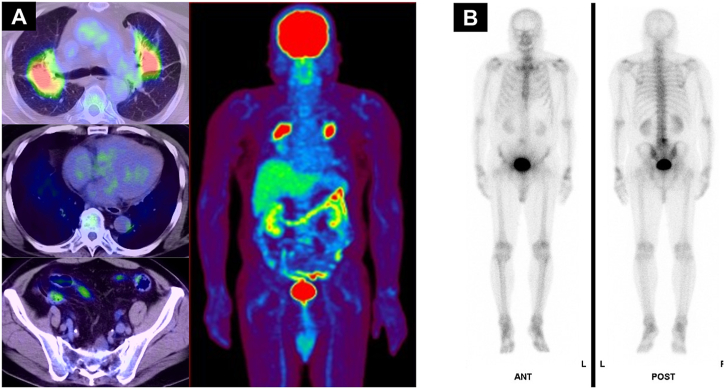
(A) After treatment, FDG-PET/CT indicated that the shadows in the lung field had decreased, and the uptake of bone lesions had almost disappeared. (B) After treatment, bone scintigraphy demonstrated decreased uptake of bone lesions.
